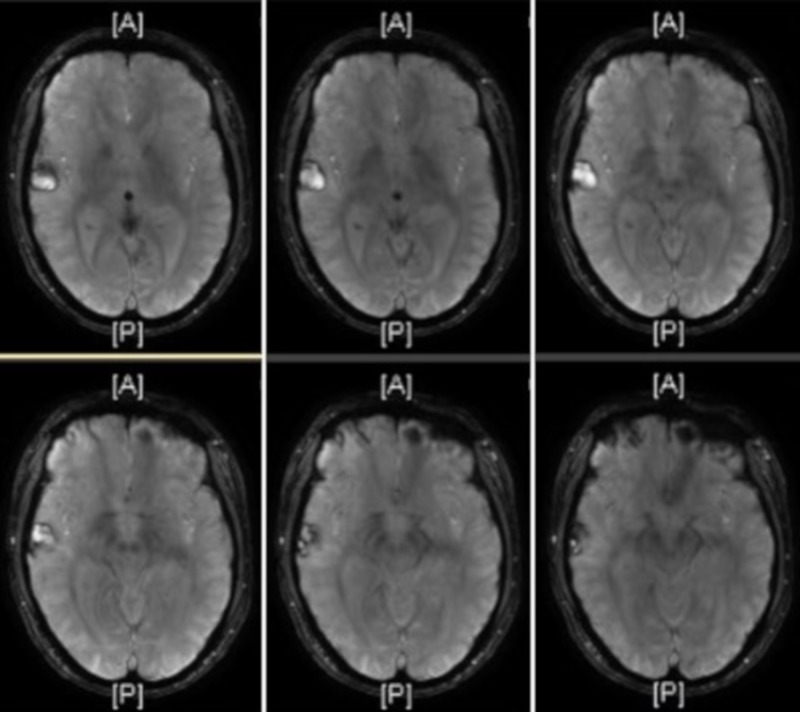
T1-weighted magnetic resonance imaging (MRI) showed several acute and subacute cerebral contusions within the right frontotemporal region with progression of the temporal lobe contusion.
